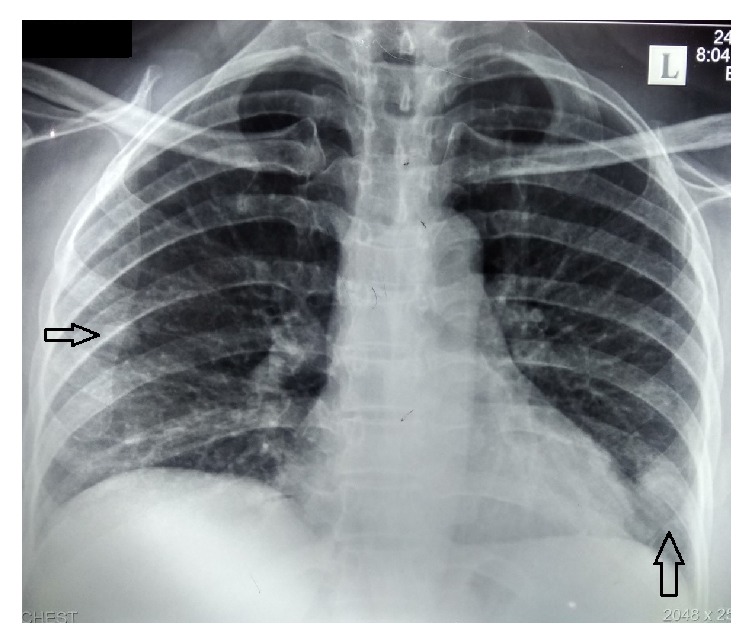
Bilateral multiple opacifications in chest radiograph.
